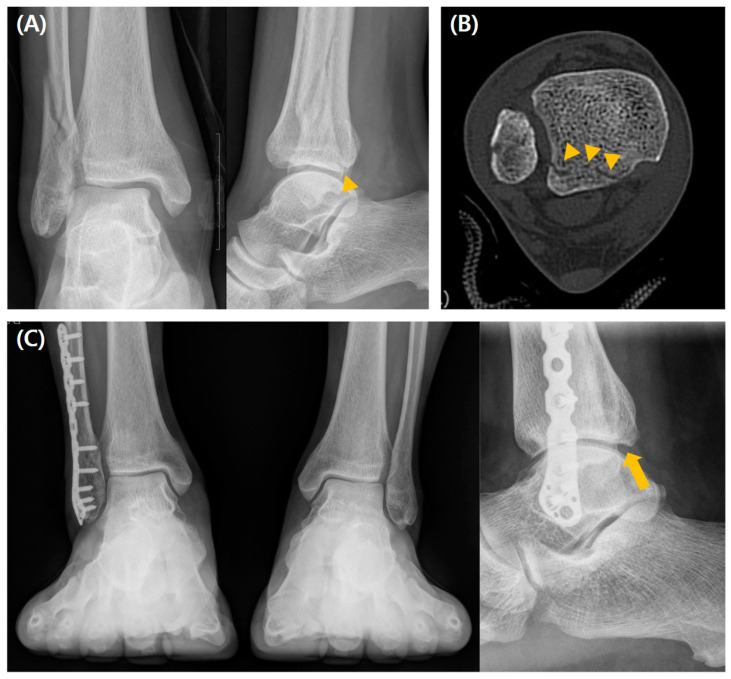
A case of a 56-year-old man who underwent conservative management for type 1 PMF. (A) Preoperative radiographs show a fracture line (arrowhead), and (B) preoperative CT scan images show a posterior malleolar fragment representing a type 1 fracture line (arrowheads) without the ICF. (C) Weight-bearing radiographs obtained 3 years postoperatively show acceptable reduction and bone healing (arrow).
